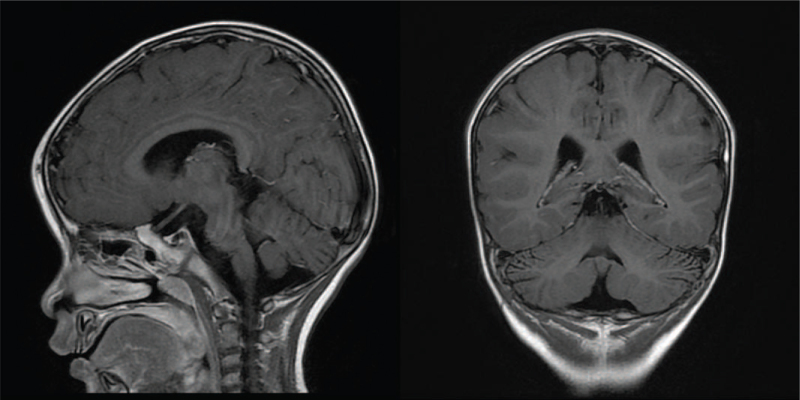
Brain magnetic resonance imaging (MRI), showing subtle sulcal widening of bilateral cerebellar hemispheres and enlargement of the fourth ventricle reflecting brain tissue loss in T1 weighted image (T1WI).
